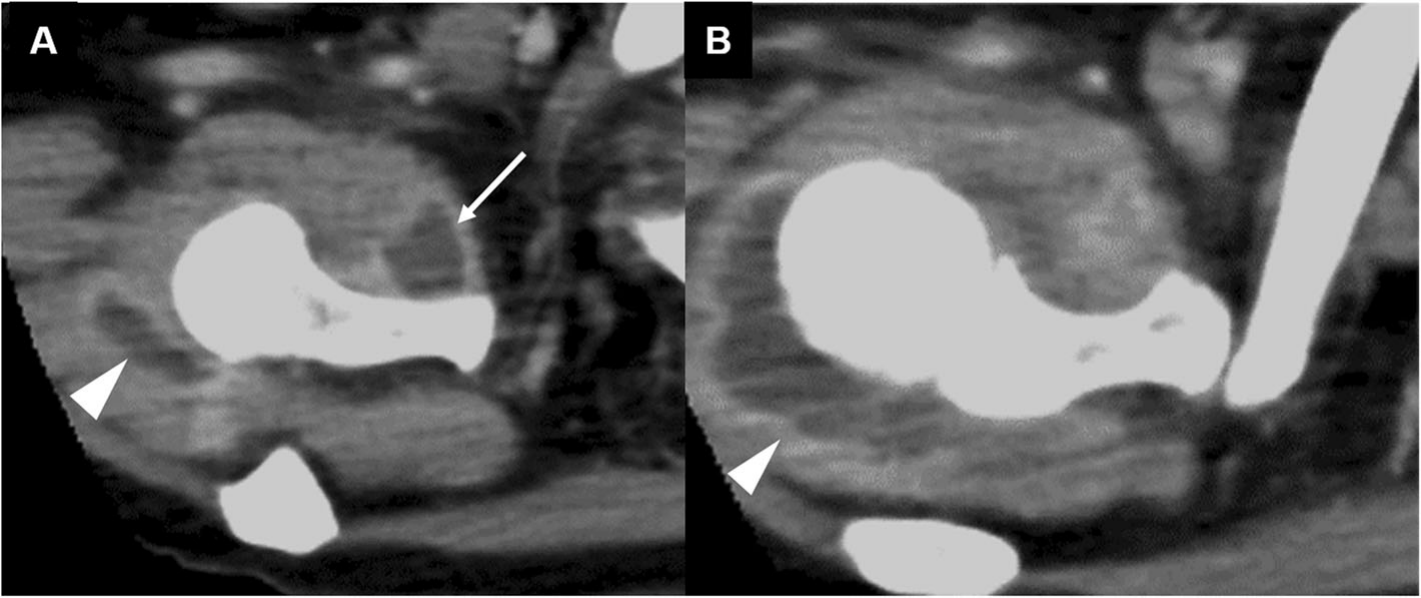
Contrast-enhanced CT imaging performed after 3 weeks of antibiotic administration revealed abscess formation in the right shoulder joint space (arrow heads), in addition to the formation of a capsule of the abscess in the right subscapularis muscle (arrow) (a and b)
